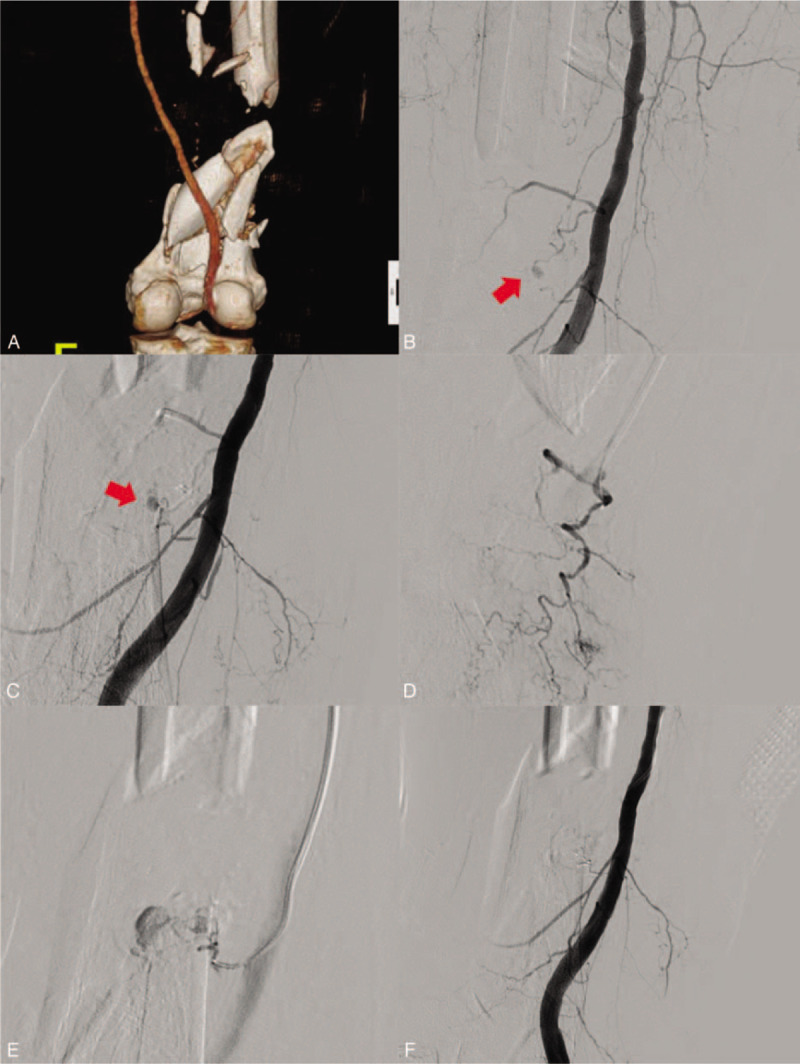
A 74-year-old man was admitted to our hospital after a car accident. (A) Initial lower extremity computed tomography (CT) revealed a comminuted fracture of the right distal femur. (B and C) Two pseudoaneurysms from a muscular branch (red arrow) and descending genicular branch (red arrow) were identified on right superficial femoral arterial (SFA) angiography. (D and E) Selective catheterization of the orifice of the bleeding branches was performed directly with a 5-F C2 catheter, and then a 1.7-F microcatheter was introduced into the proximal portion of the bleeding branch using a coaxial technique. Two bleeding branches were embolized with 1:2 mixtures of *n*-butyl cyanoacrylate (NBCA) and iodized oil. (F) A post-embolization angiography demonstrated successful hemostasis, with no complications.
